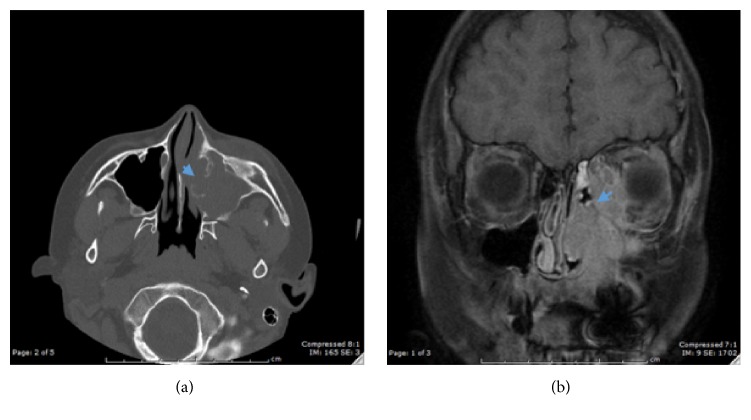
Image of mass in left anterior ethmoid cell extending into left medial aspect of orbit on axial sinus CT without contrast (a), and MRI orbit T1 after contrast (b). Blue arrow indicates area of bony destruction.
